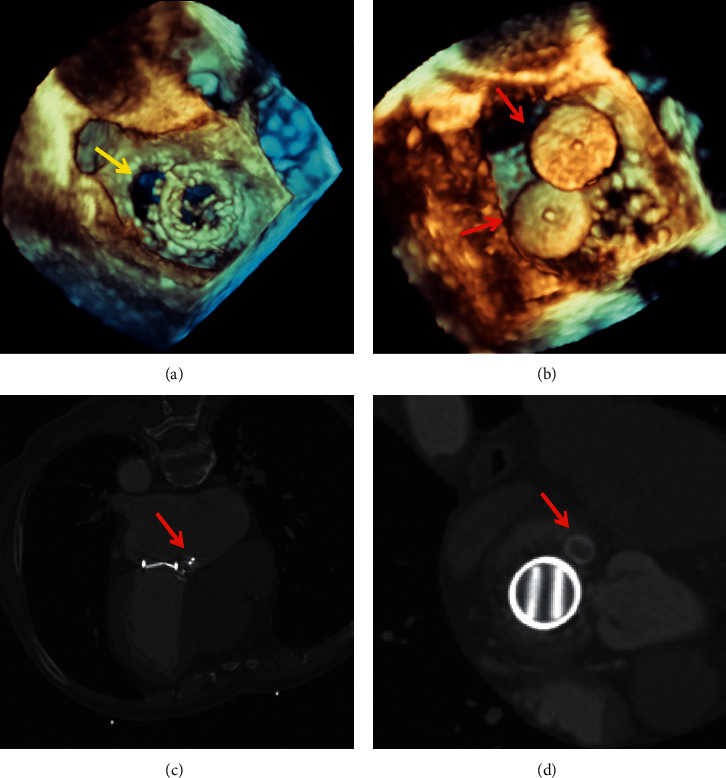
The 3-dimensional echocardiograms and computed tomography angiograms taken before the procedure and during the follow-up period. (a) Three-dimensional transesophageal echocardiography shows the mitral paravalvular leak (PVL) before the procedure. (b) Three-dimensional transesophageal echocardiography shows the mitral PVL closed with the occluder. (c) The mitral PVL was closed with the occluder (sagittal view). (d) The mitral PVL was closed with the occluder (axial view). The yellow arrow indicates the PVL. The red arrow indicates the occluder.
